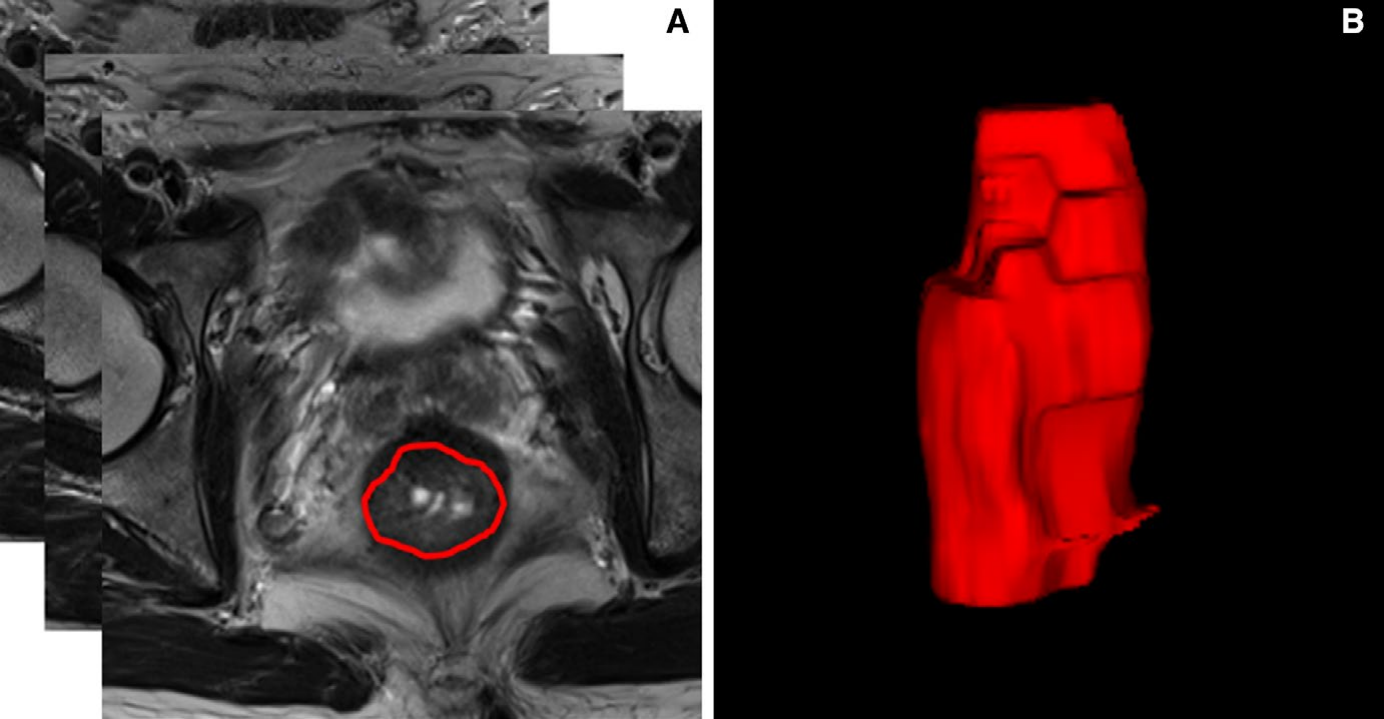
Example image for rectal cancer contouring. A, The outline of regions of interest on one slice of axial T2‐weighted MR image. B, Volume rendering
